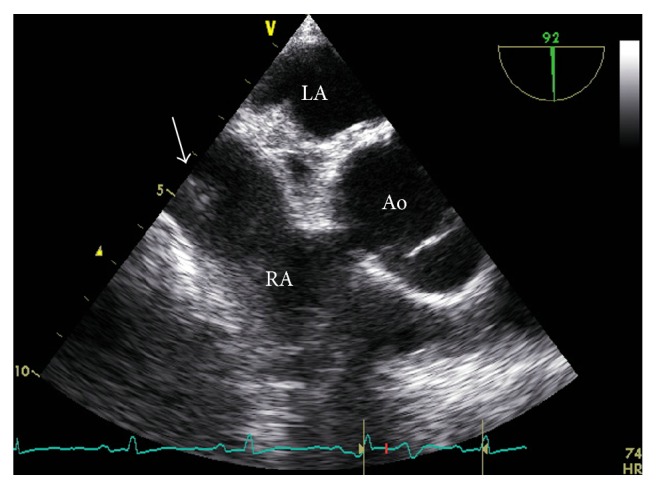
The postprocedural transesophageal echocardiogram (TEE) findings showed the residual floating structure in the RA (arrow), suggesting the tissue of Chiari's network. Ao: ascending aorta, LA: left atrium, and RA: right atrium.
